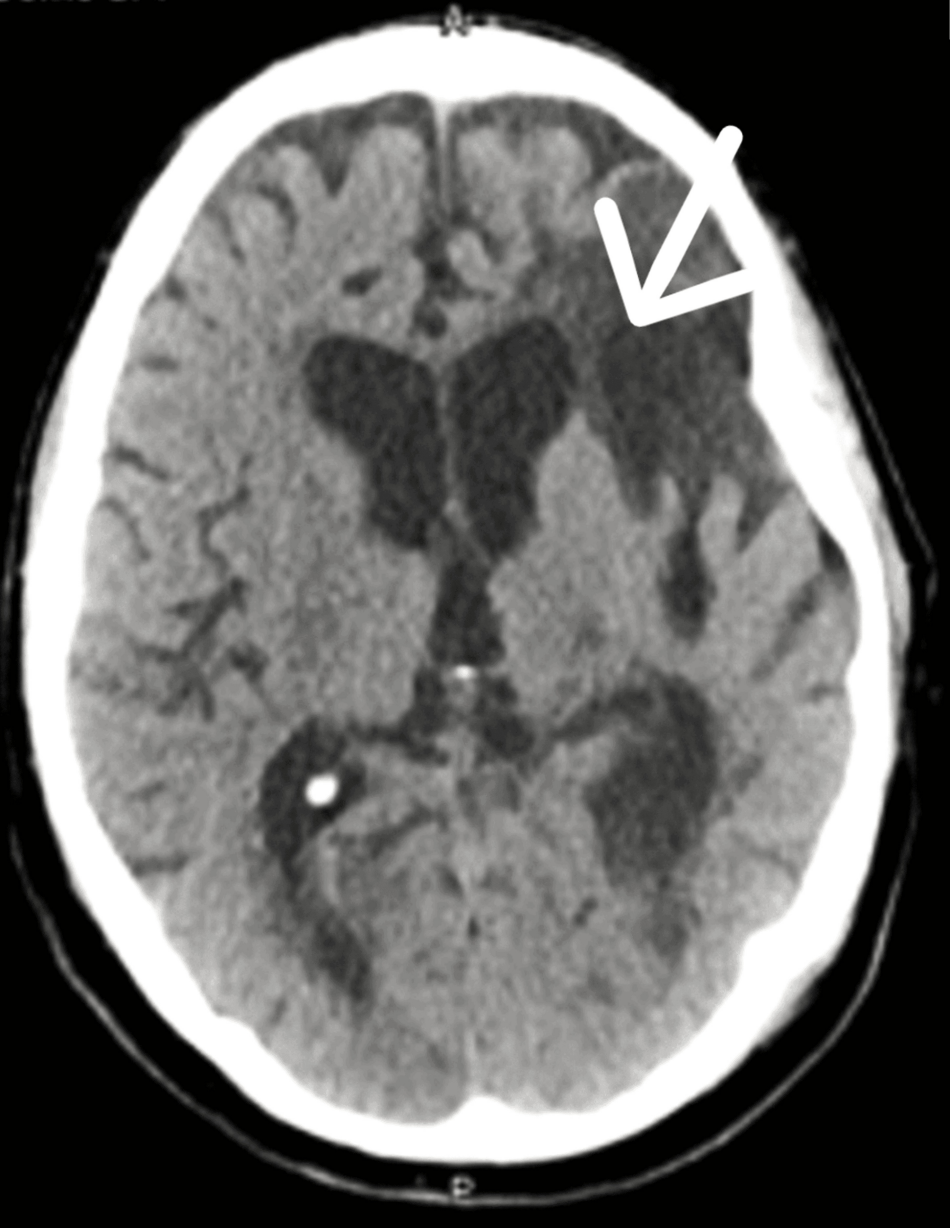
CT scan brain after one year
